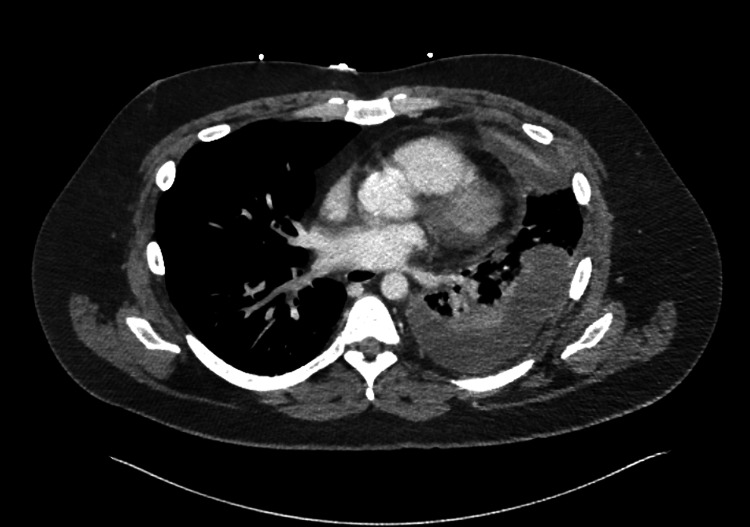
CT scan showing large left pleural effusion/empyema before chest tube placement
